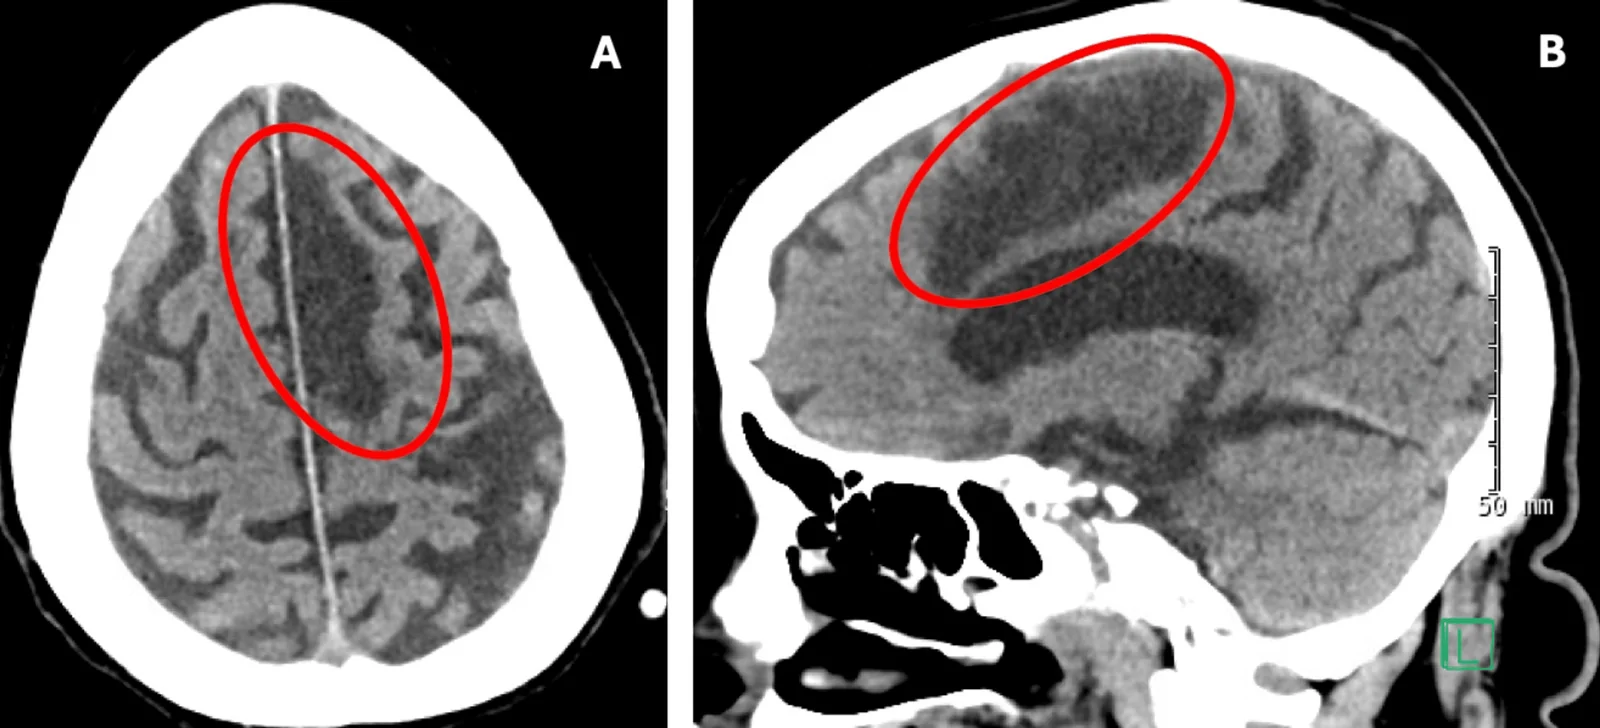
CT head without contrast revealing a large area of hypoattenuation in the left paramedial frontal lobe (red circle) in the (A) axial view and (B) sagittal view. When compared to a prior CT head, this was concerning for evolution of a prior ischemic stroke
